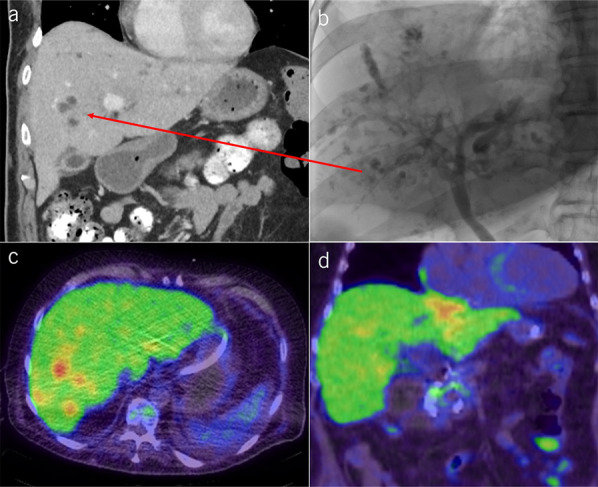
Liver abscesses in post–COVID-19 cholangiopathy. (a) CT of a 52-year-old man confirmed abscesses that were suspected in the ERCP 1 day before. (b) Same patient as in A: ERCP showed multiple small abscesses in the right hepatic lobe, the patient required LT. (c) Axial positron emission tomography-computed tomography showed multiple liver abscesses in a 60-year-old man with post–COVID-19 cholangiopathy. (d) Same patient as in C: frontal plane showed an abscess in the left liver lobe extending to the left ventricle. The patient did not survive. COVID-19, coronavirus disease 2019.
